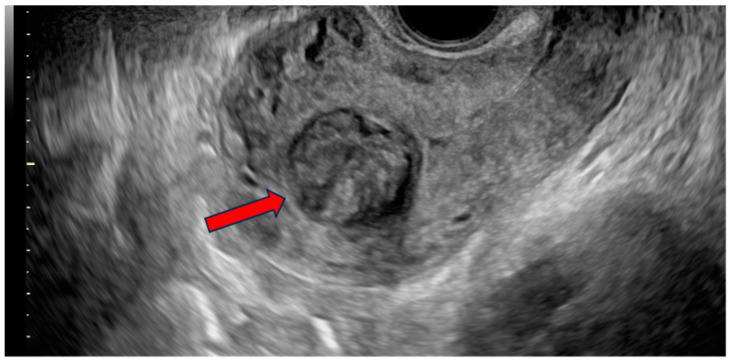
Transvaginal ultrasound (TVS) image showing a sagittal view of the patient’s uterus. A well-defined, solid lesion (red arrow) of mixed echogenicity is visualized within the borders of the uterine cavity. No evident acoustic shadow is present. The myometrium surrounding the lesion displays visible heteroechogenity with rich vascularization.
